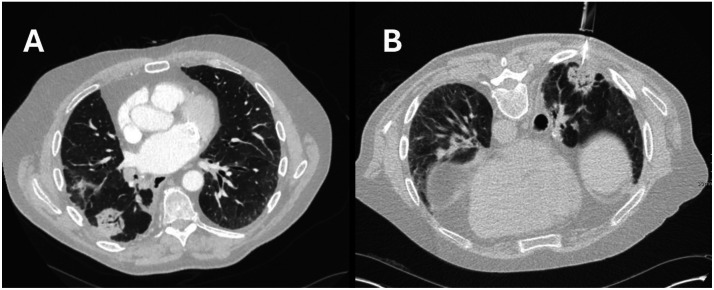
CT scan lung view (A) right middle lobe consolidation (B) biopsy needle.
